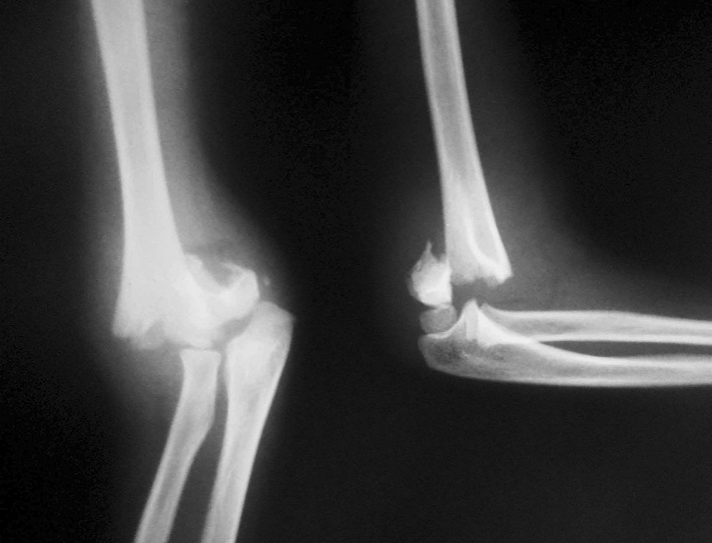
Radiograph of the right elbow in a 6-year-old boy: anteroposterior and lateral views showing displaced extension type supracondylar fracture of the humerus
